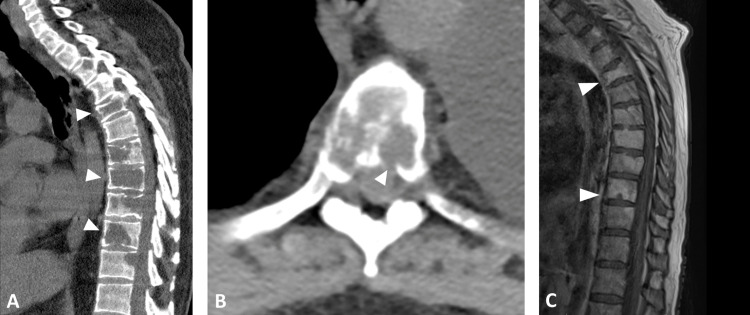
A. CT of thoracic spine, sagittal view, with white arrows depicting metastases. B. CT of thoracic spine, axial view, with white arrows depicting metastases. C. T1-weighted, post-contrast MRI image of the thoracic spine with white arrows depicting metastases. CT, computed tomography; MRI, magnetic resonance imaging.
